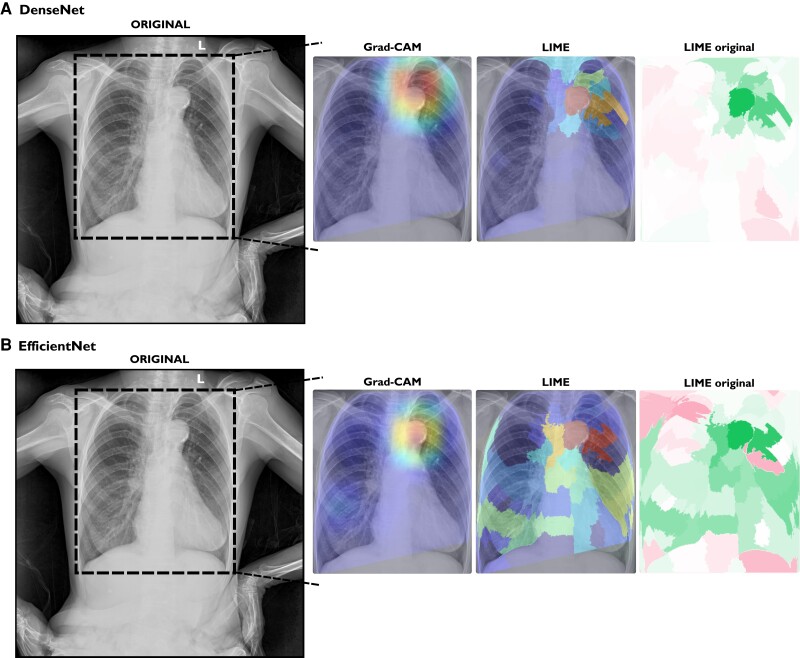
Explainable methods’ results for an exam correctly labelled as aortic elongation for both models. (*A*) DenseNet prediction probability of aortic elongation: 99.44%. (*B*) EfficientNet prediction probability of aortic elongation: 99.64%. In the original image, a region of interest is demarcated by a dashed box. The output of each method, gradient-weighted class activation mapping and local interpretable model-agnostic explanations, is superimposed on the chest X-ray image. The most relevant regions are highlighted in red, while the least relevant regions are indicated in blue. The ‘LIME original Mask’ refers to the mask generated by the local interpretable model-agnostic explanations method. Grad-CAM, gradient-weighted class activation mapping; LIME, local interpretable model-agnostic explanations.
